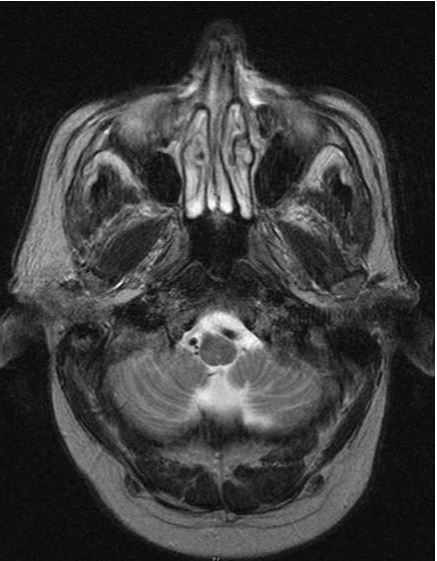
MRI (axial): Left infraorbital mass with infiltration to the lateral nasal root.
